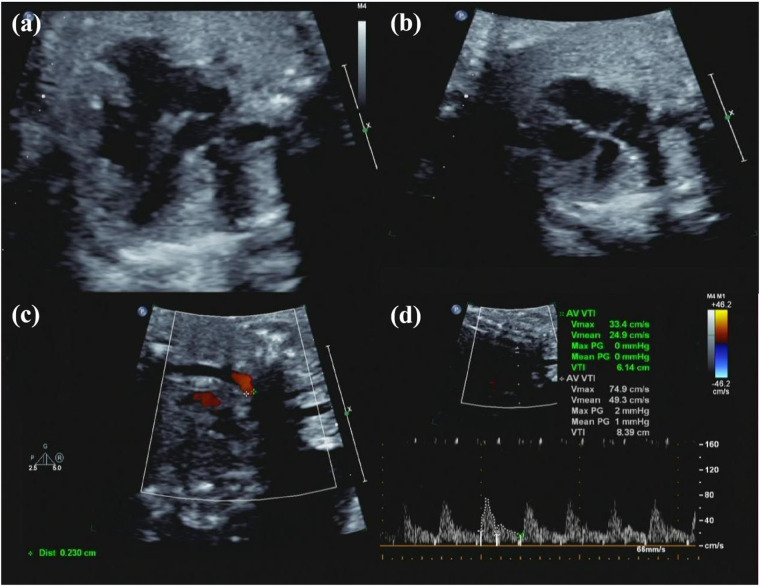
Prenatal fetal echocardiographic images. (a) No obvious SVAS was detected prenatally; (b) LRCCD was observed in the four-chamber view; (c) Mild narrowing of the aortic isthmus was noted; (d) accelerated flow with diastolic flow was observed in the aortic isthmus.
